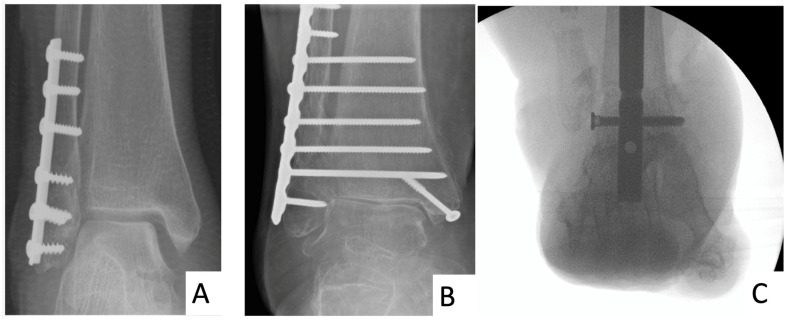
Radiographs of standard open reduction–internal fixation (A), extended open reduction–internal fixation (B) and a tibio-talar-calcaneal (hindfoot) nail (C).
